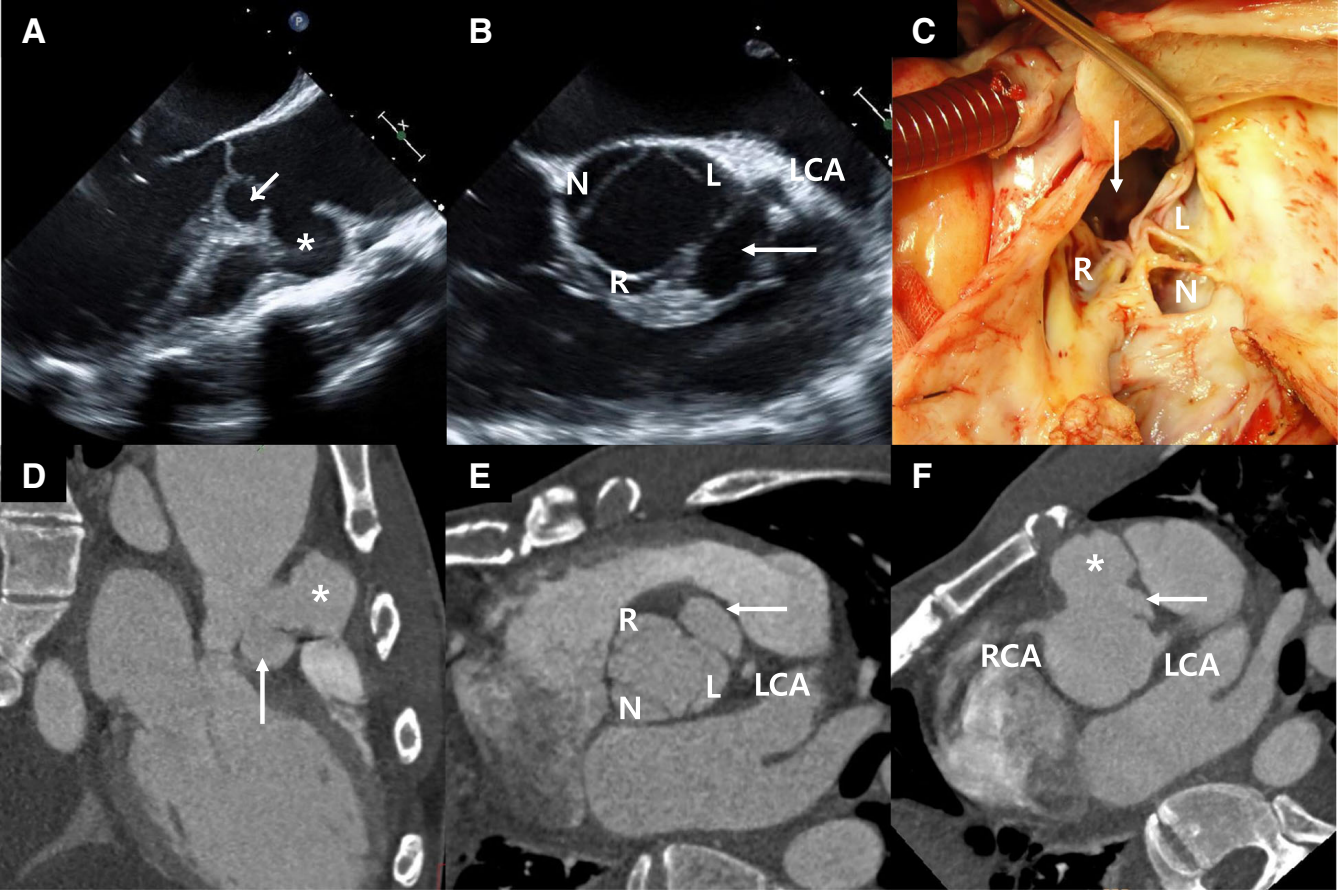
Representative case of Behcet’s disease-related aortic regurgitation. A 36-year-old male presented with Behcet’s disease-related severe aortic regurgitation. Pseudoaneurysm (asterisk) and interventricular septal dissection (arrow) between the right and left coronary cusps were clearly shown on transesophageal echocardiography (a, b), in the surgical field (c) and on computed tomography (d-f). R, right coronary cusp; L, left coronary cusp; N, non-coronary cusp; LCA, left coronary artery; RCA, right coronary artery
